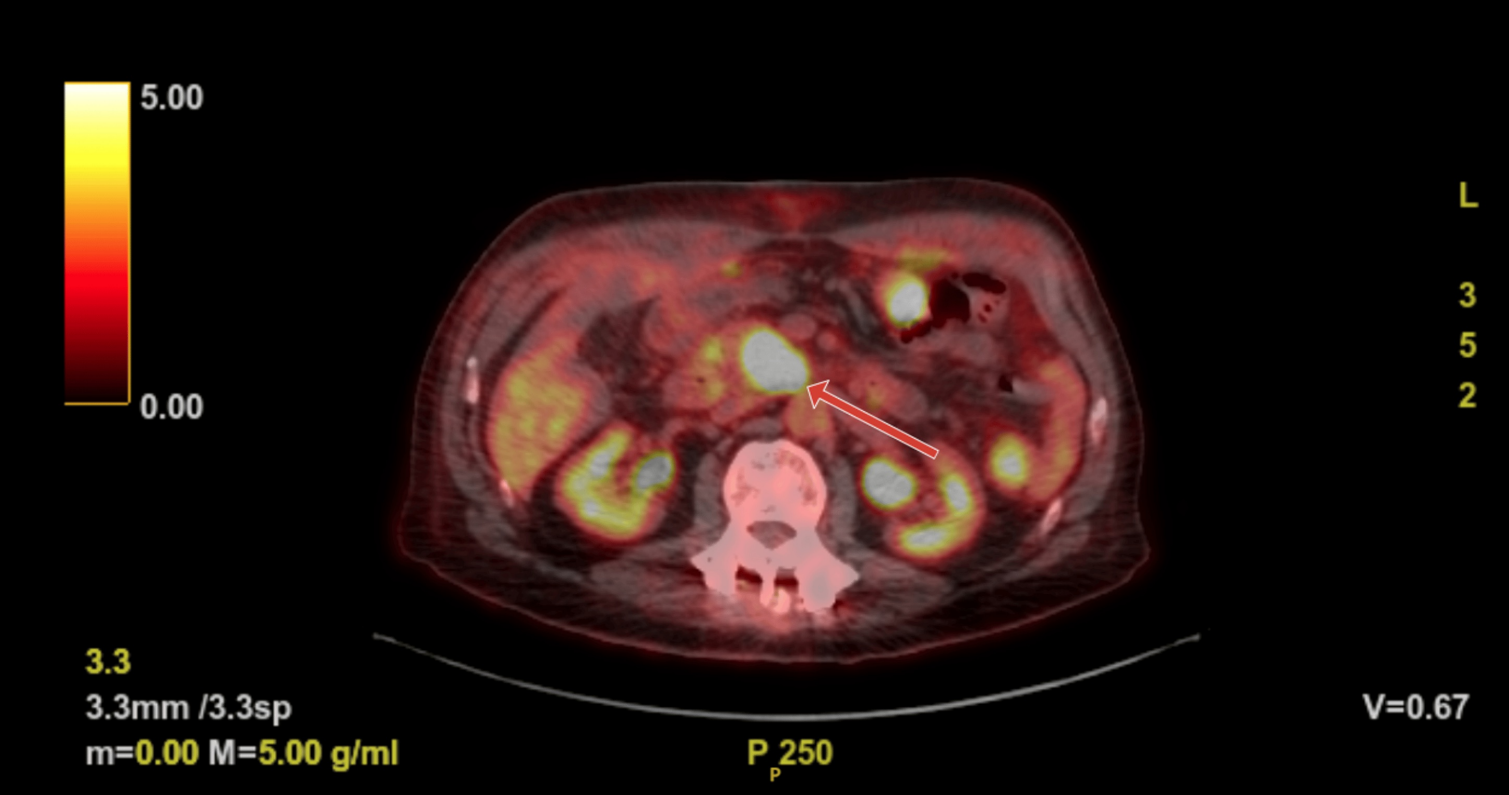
PET-CT, with red arrow pointing to hypermetabolic head of pancreas mass.
